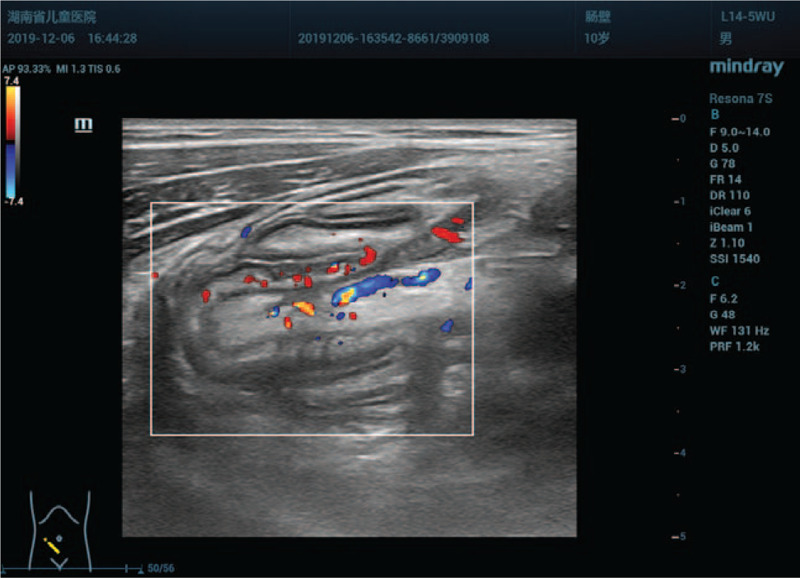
Longitudinal view of the intussusceptum shows a finger-like appearance, and color Doppler flow imaging shows an artery supplied to the intussusceptum.
